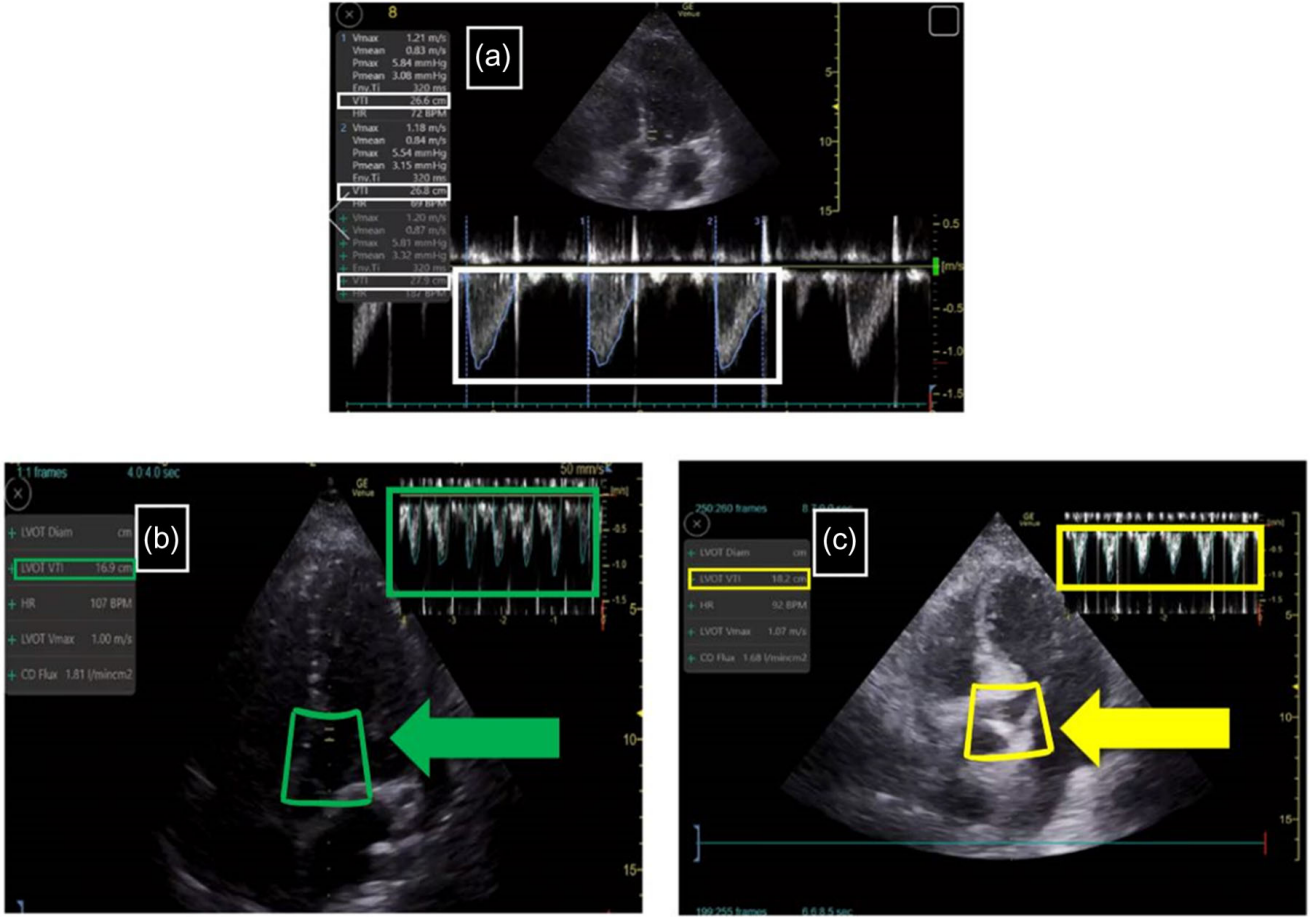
Manual LVOT‐VTI and auto LVOT‐VTI acquired by ICU doctors. (2a) Manual LVOT‐VTI measurements by ICU doctors in apical five‐chamber view, the large white box showed three cycles of manual LVOT‐VTI and the small white box indicated the exact value of each manual LVOT‐VTI. (2b) AI‐VTI tool screen layout of ideal view, the left green box displayed the auto LVOT‐VTI value calculated by AI software instantly, the middle green box of ROI and the green arrow emphasized the ideal quality of the image, the green box in the upper right corner contained all the cycles that AI calculated. (2c) AI‐VTI tool screen layout of average view, the left yellow box displayed the AI‐calculated auto LVOT‐VTI, the middle yellow box of ROI and the yellow arrow indicated the average quality of the image, the left box in the upper right corner contained all the cycles that AI calculated
